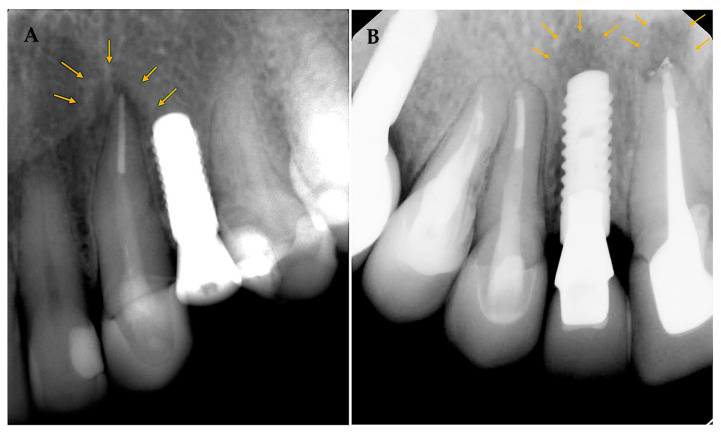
(A,B) show implant cases with RPI. Arrows to outline the periapical lesions in relation to teeth and adjacent implants.
